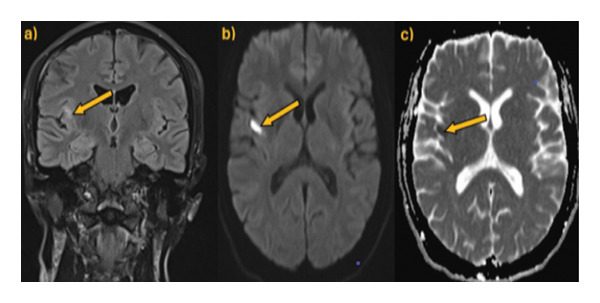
Brain MRI showed acute embolic ischemic lesions in the right insular and frontal juxtacortical regions, pointed with arrows: (a) hyperintensity on axial FLAIR, (b) hyperintensity on axial DWI, and (c) hypointensity on the axial ADC map.
